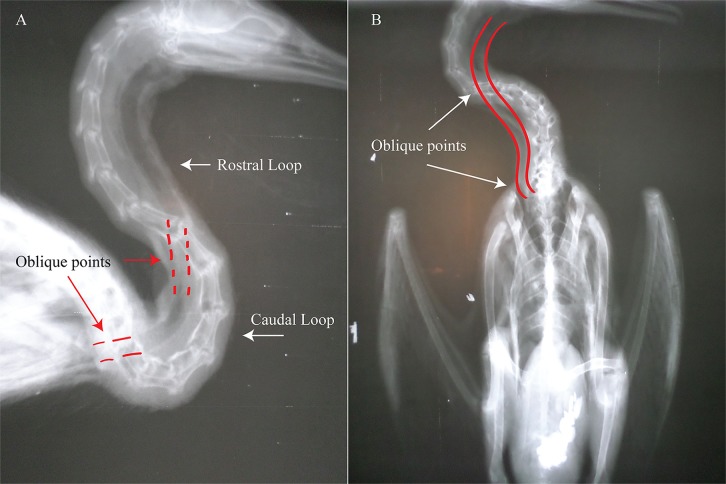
Radiographs of *P*. *auritus* neck. (A) “Rostral loop” is the upper half of the “S” shape of the bird neck; while the “caudal loop” is the lower half. Terminology after Van der Leeuw et al. [63]. Lateral view showing the course of the trachea and its oblique points (point where the trachea crosses planes (medial to lateral and vice versa). (B) ventral view showing the course of the trachea and its oblique points.
